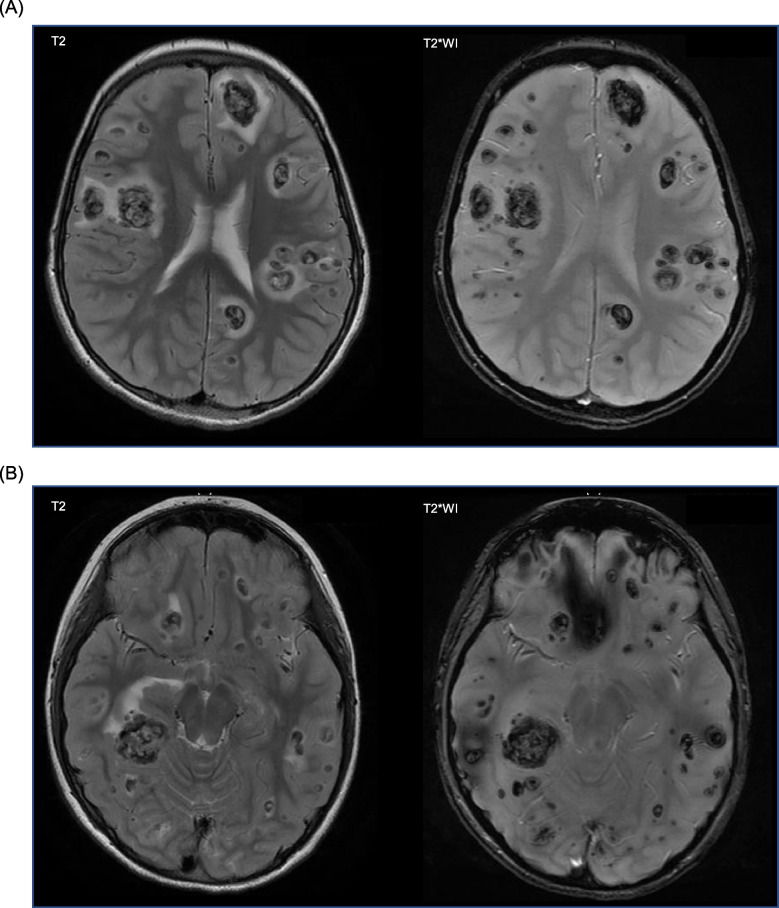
Multiple central nervous system infiltrations with hemorrhages on magnetic resonance images (MRI). A and B T2-weighted and T2*-weighted image (T2*WI) MRI shows heterogeneous signals with leukemic masses and hemorrhages. Edema is observed around multiple lesions
